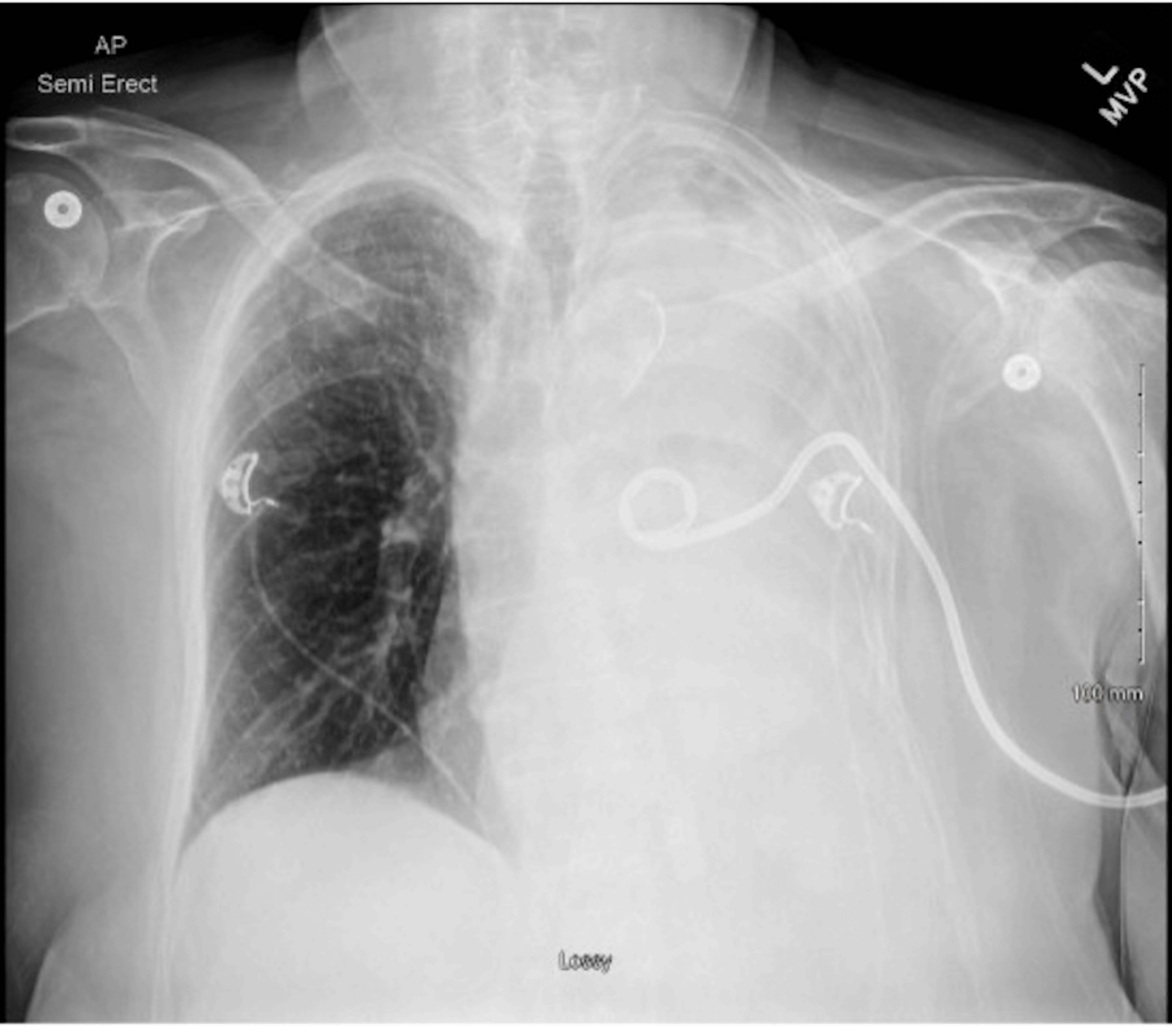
Anteroposterior (AP) chest x-ray showing complete opacification of the left hemithorax. The image indicates a significant pleural fluid accumulation consistent with hemothorax. A left-sided pigtail pleural catheter is also visible, confirming the placement.
